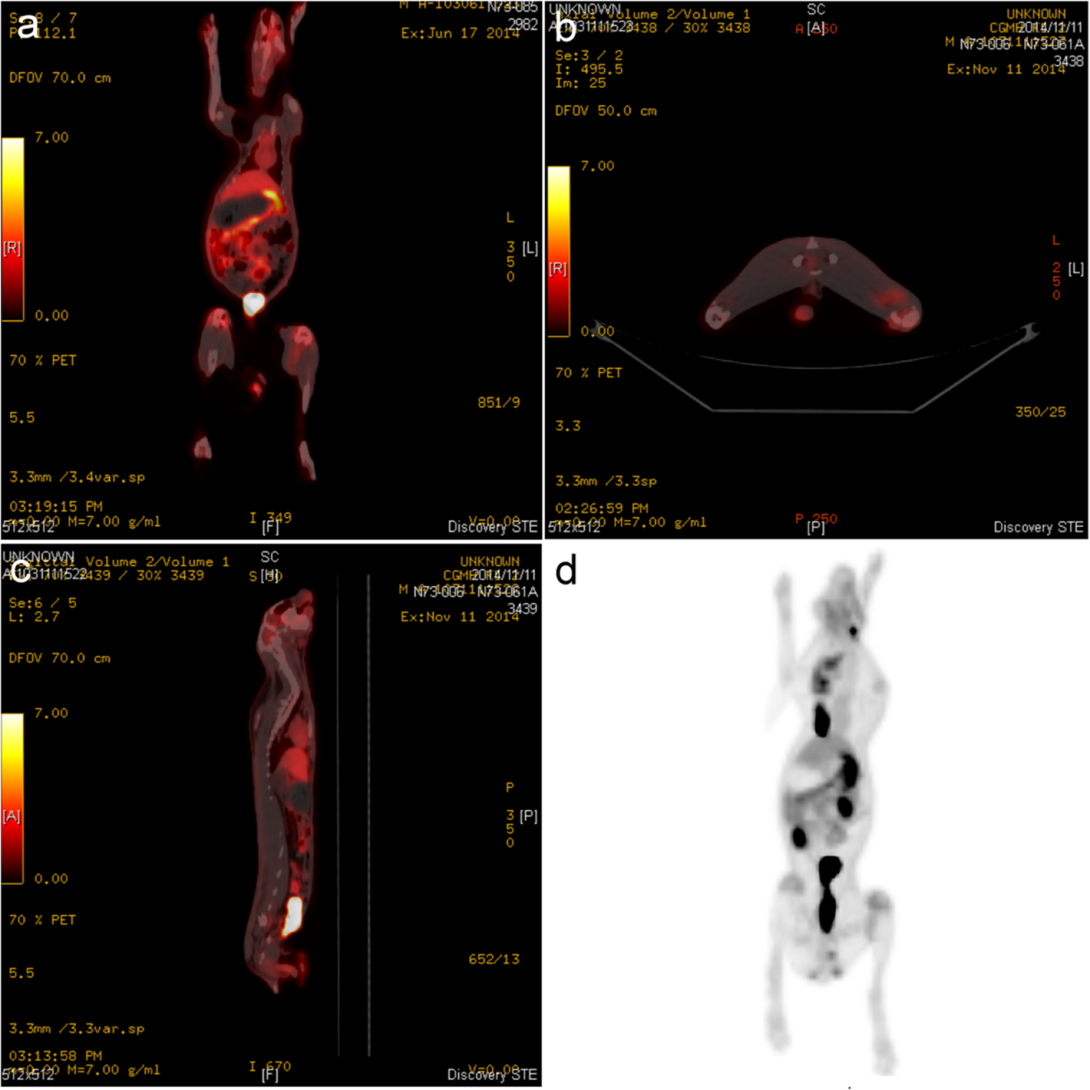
^18^F-FDG PET/CT imaging. Coronal (a), transaxial (b), and sagittal (c) ^18^F-FDG PET/CT images. (d) PET image for quantitative analysis
